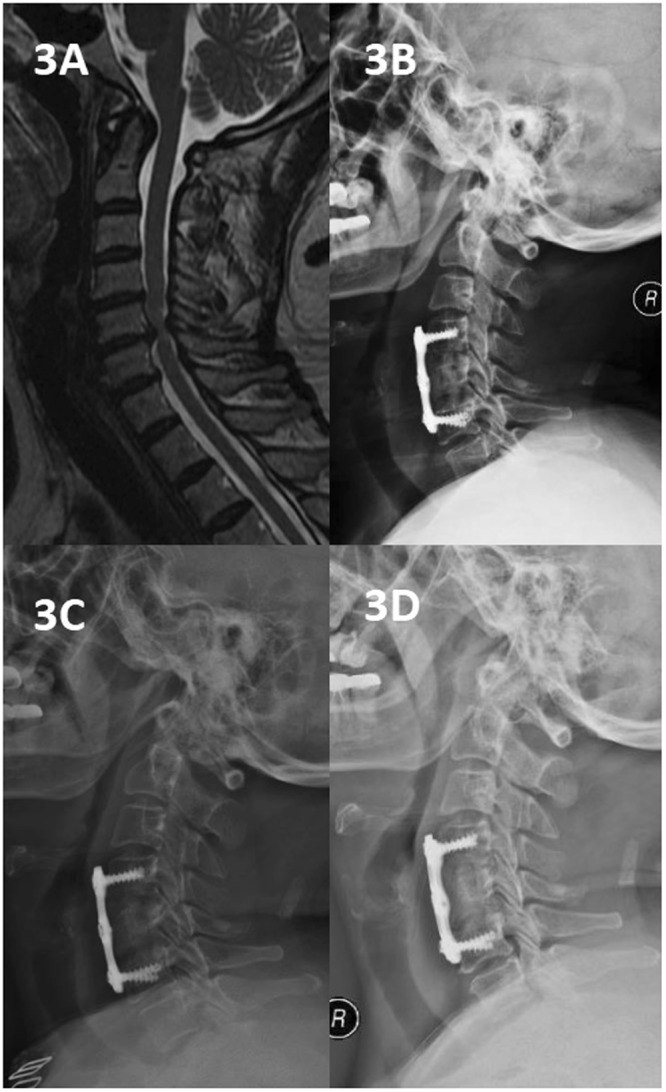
A 60-year-old woman who underwent 1-level corpectomy with a nanohydroxyapatite/polyamide66 strut for cervical reconstruction. The preoperative cervical MRI (A) revealed C5/6 disc herniation. The patient underwent a C5 corpectomy and fusion using an n-HA/PA66 strut (B). The lateral X-ray film indicated that the autogenous bone granules filling the strut had achieved bony fusion, and subsidence was observed at the 6-month follow-up (C). The strut and internal fixation were in position at 3, 6, and 12 months of follow-up (B–D).
